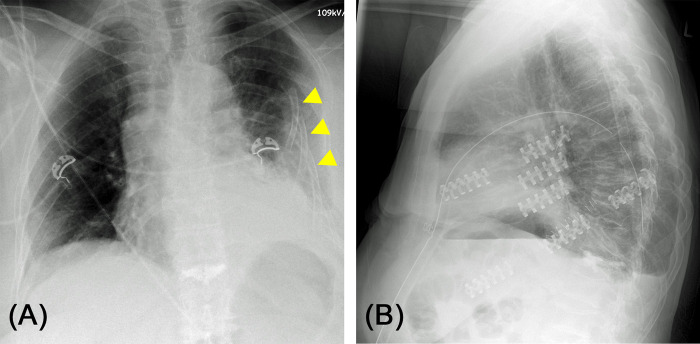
(A) Chest X-ray of a 73-year-old female patient after home accident. Significantly dislocated serial rib fracture of the left side with involvement of all ribs except the 12th rib. Several ribs with multiple fractures causing free fracture segments (“floating rib”). (B) Post-operative lateral chest X-ray after osteosynthesis of costae 4 to 7. Not all fractures needed to be fixated to achieve stabilization of the chest wall and regain physiological contour of the thorax.
